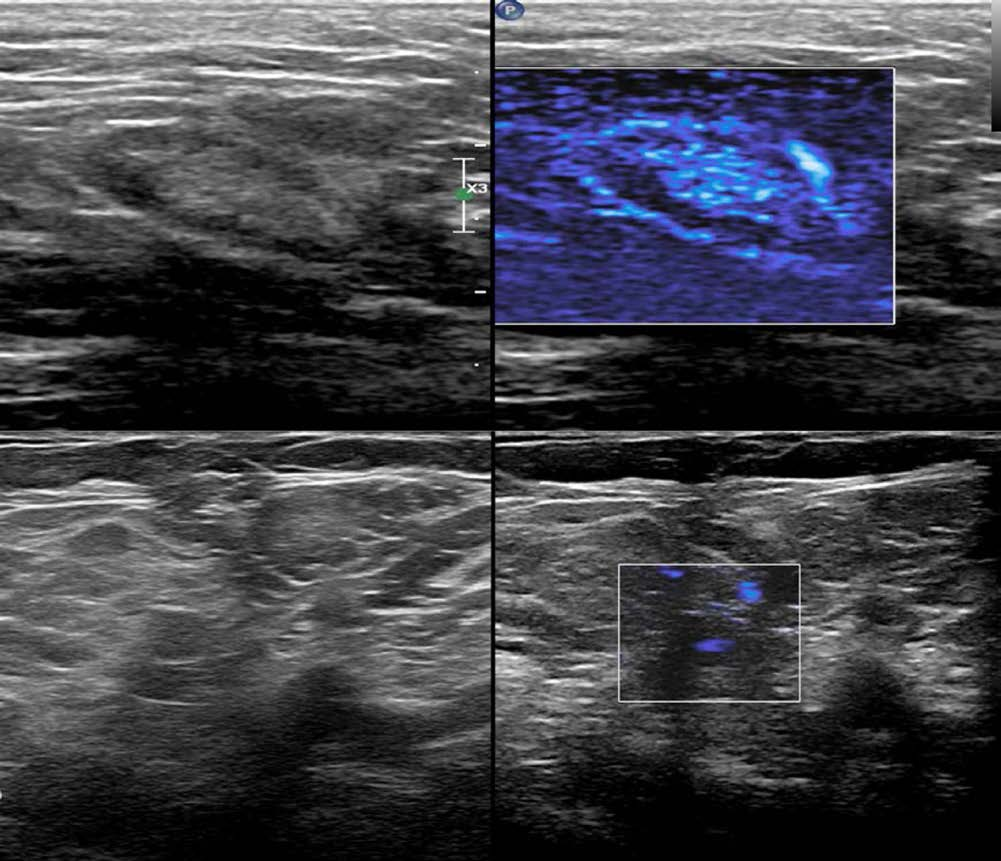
Ultrasonography, showing axillary and inguinal lymph nodes were oval and had normal hilums.
